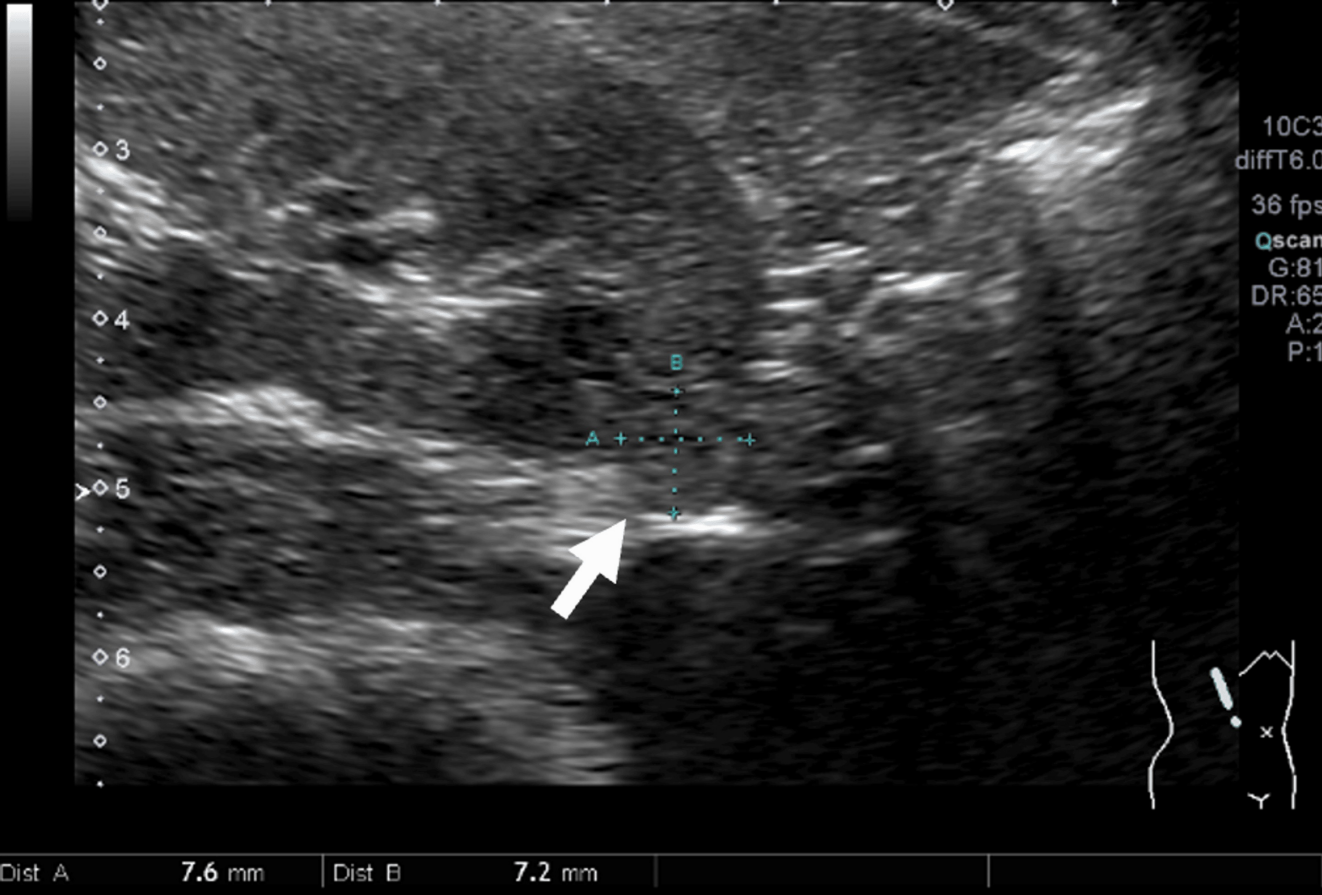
Abdominal ultrasonography performed four months before pregnancy A small renal mass, suspected to be renal cell carcinoma, is seen in the right kidney on abdominal ultrasonography.
